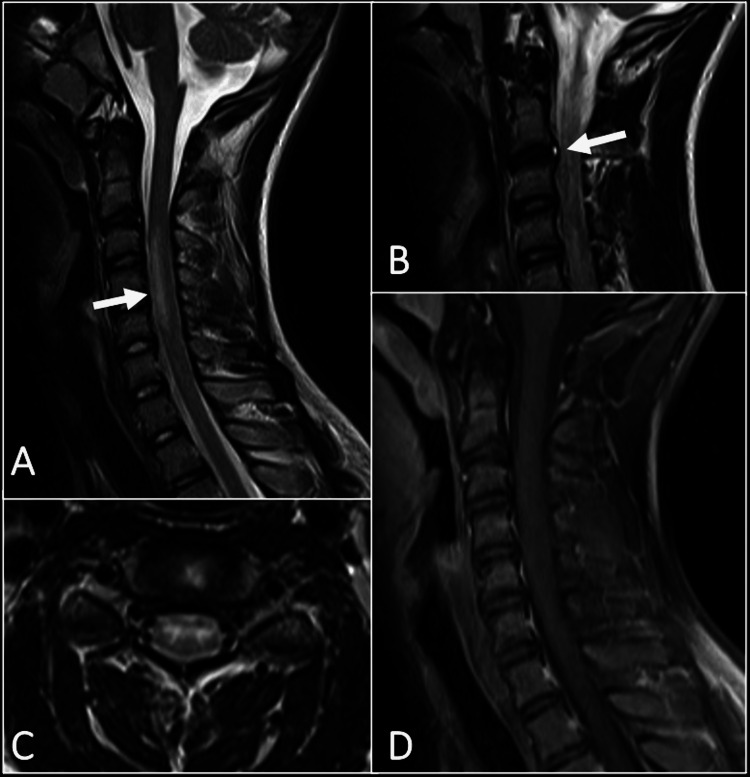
Initial MRI: (A) Sagittal T2 weighted image of the cervical spine shows intramedullary signal abnormality from C3 to C5 in the anterior cord with mild swelling of the cord (arrow). (B) There are isolated reduced signal intensities of the intervertebral disc at C2-C3 with posterior hyperintensity in keeping with the annular tear (arrow). (C) The axial T2 image of the cord at C3-C4 shows that the signal abnormality is involving the central gray matter. (D) No abnormal enhancement is seen in the cord in this post-contrast image.
